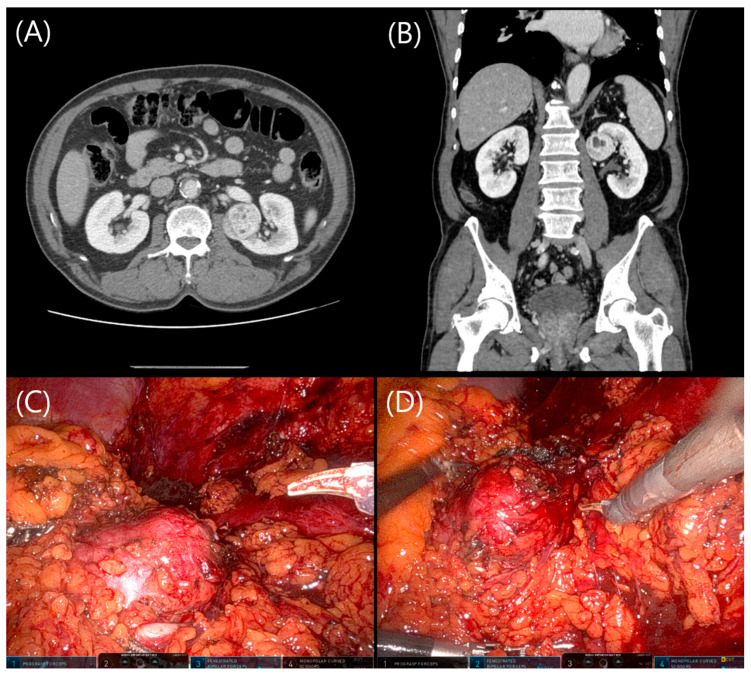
Preoperative and intraoperative imaging of a left renal tumor located at the postero-medial upper pole. (A,B) Axial and coronal views of abdominal CT show a well-defined enhancing mass at the upper pole of the left kidney, primarily located on the posterior and medial aspect. (C) Standard transperitoneal view during robotic partial nephrectomy demonstrates limited visualization of the posterior tumor surface. (D) After camera port swapping, improved visualization is achieved, allowing better exposure and instrument access for tumor excision.
